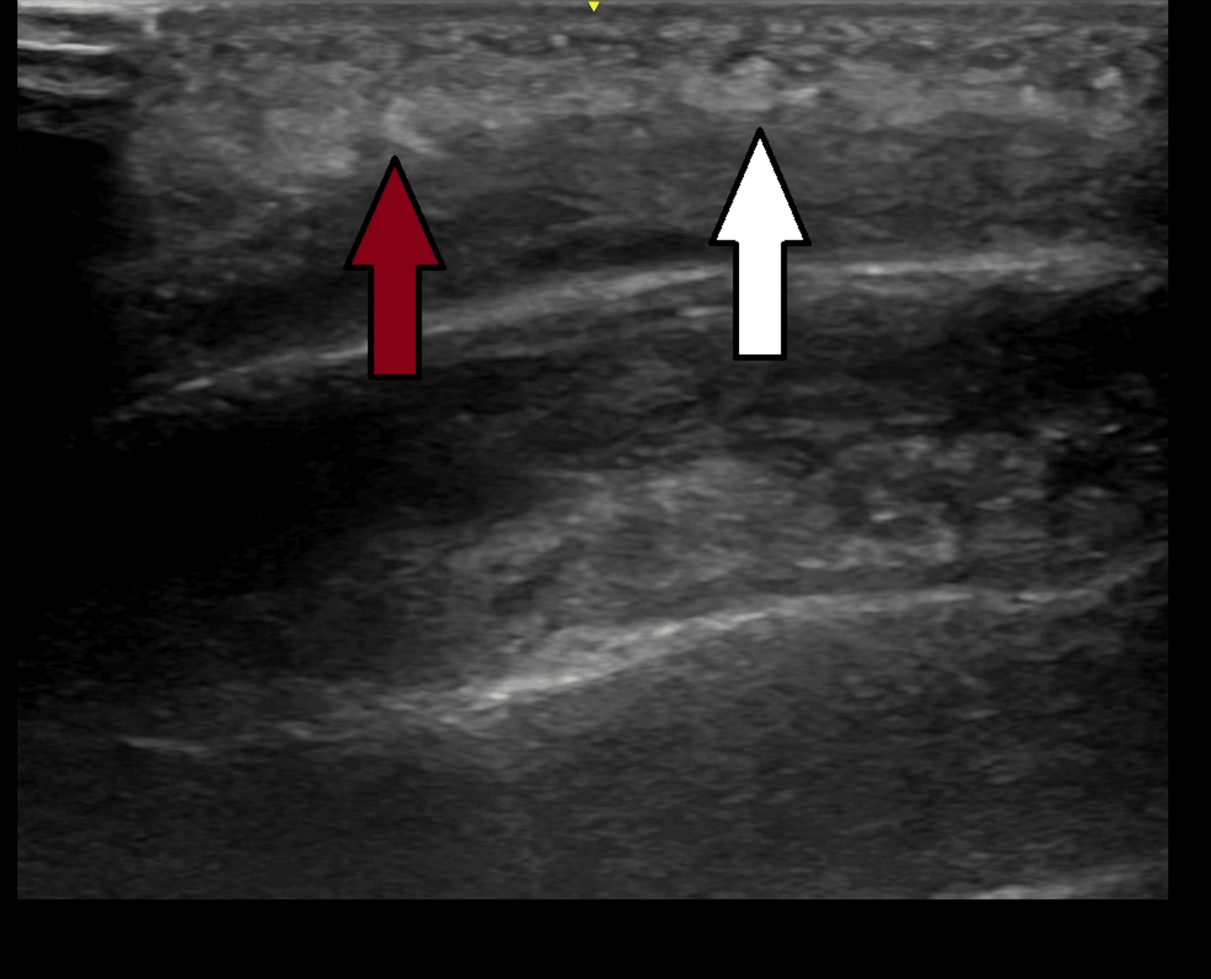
POCUS finding of cobblestone pattern with soft tissue swelling. The red arrow points to the soft tissue swelling, and the white arrow demonstrates a cobblestone pattern observed in the point-of-care ultrasound (POCUS) of the forehead swelling.
